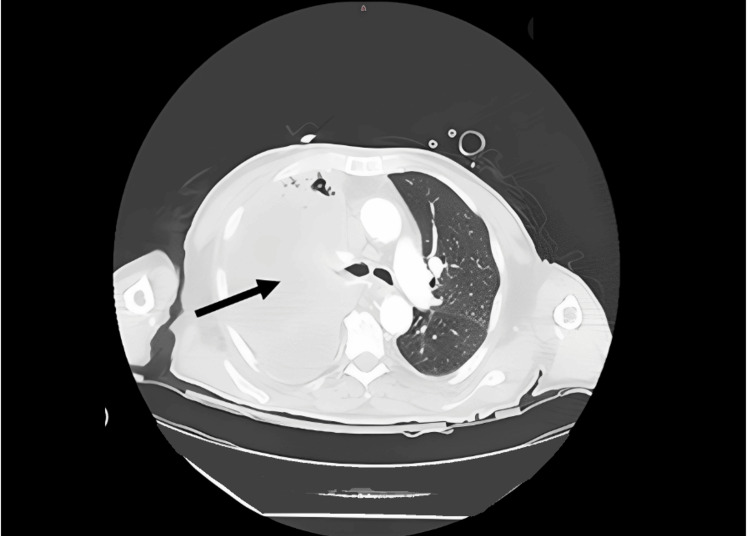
Thoracic CT. The black arrow indicates right main bronchus obstruction with secondary lobar atelectasis and pleural effusion.
